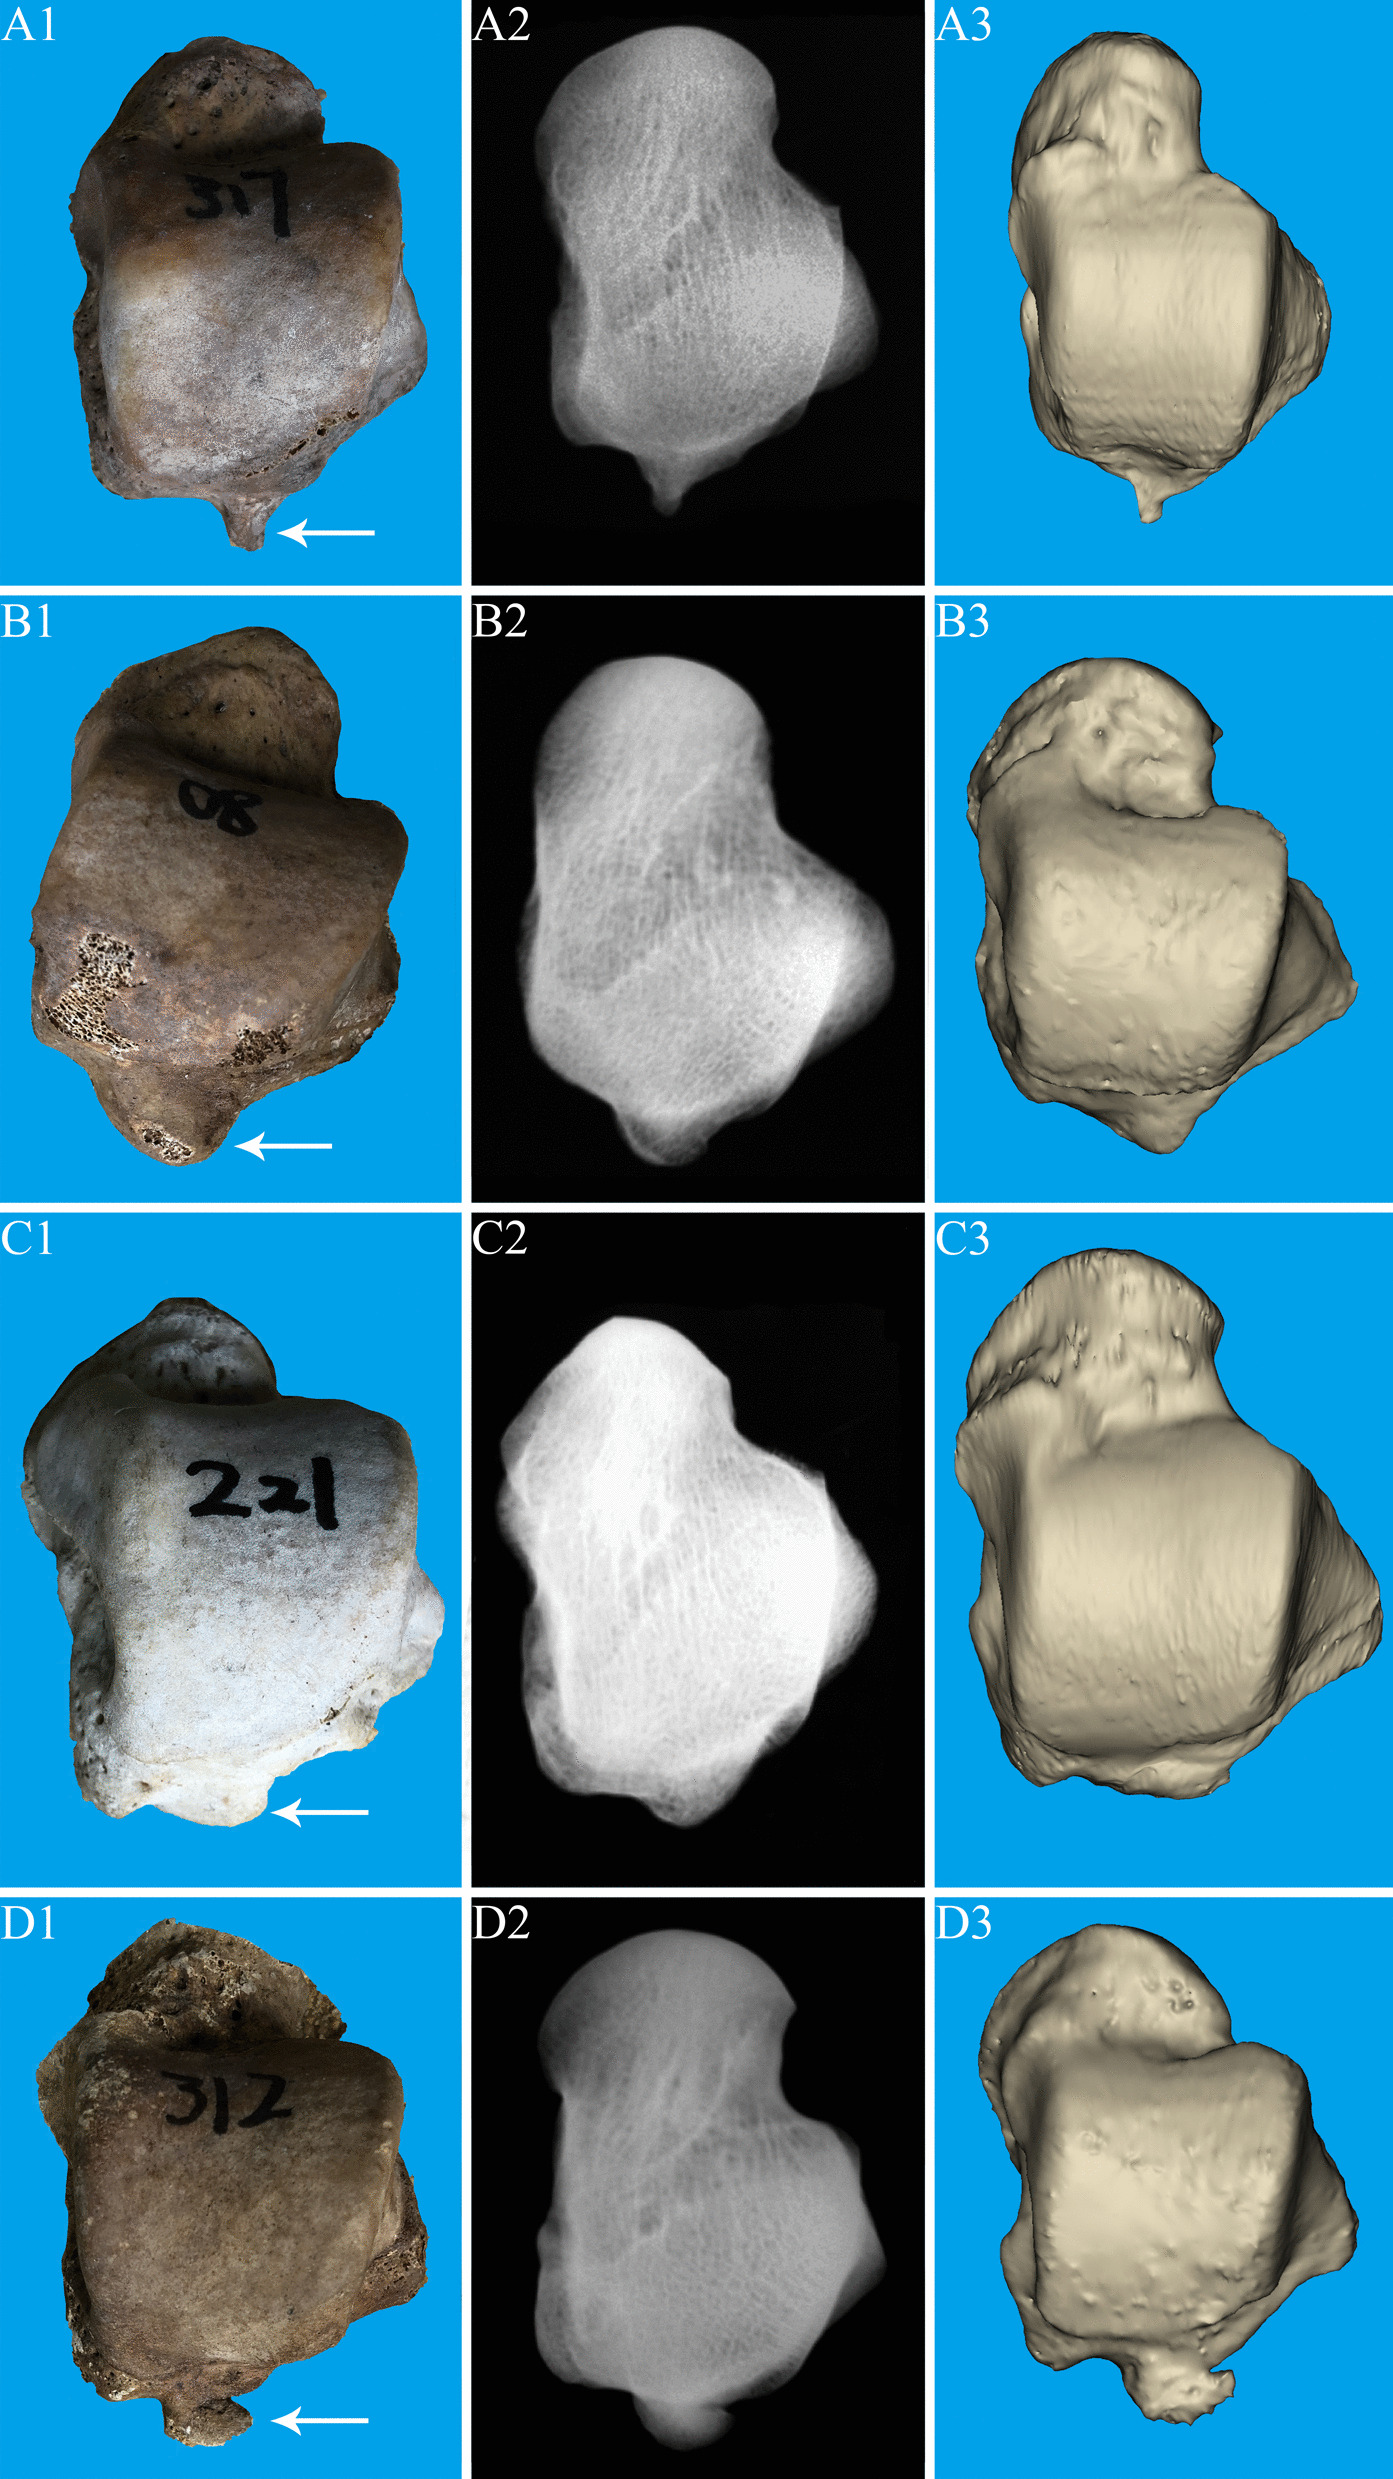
The types of the posterior process of the talus (anatomy, X-ray and three-dimensional reconstructed image). A1–A3 Type 1: pointy type; B1–B3 Type 2: round blunt type; C1–C3 Type 3: flat type; D1–D3. Type 4: neck-like type
